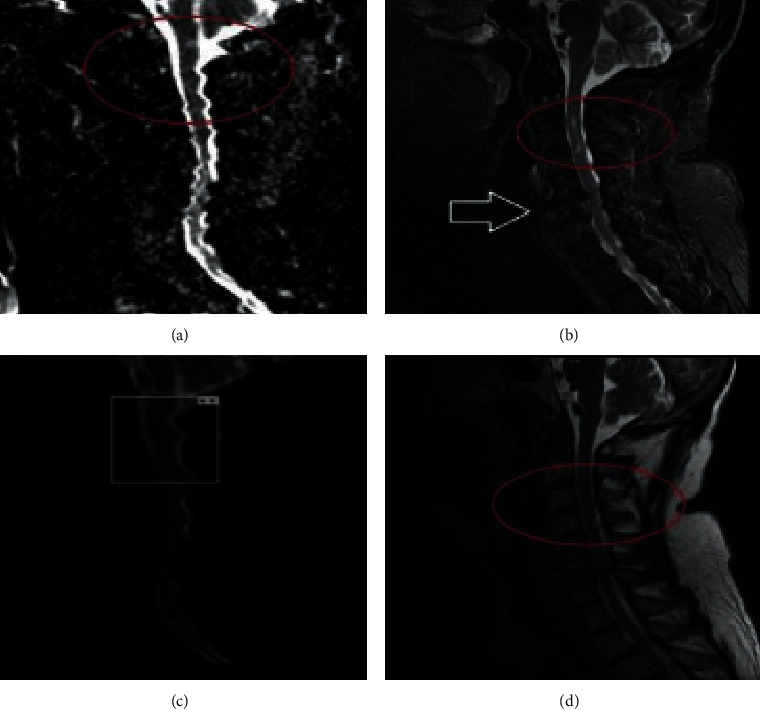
(a–d) MRI of the cervical spine with and without gadolinium demonstrated a restriction on diffusion-weighted imaging (DWI) (a) corresponding to the signal abnormality foci seen on short-TI inversion recovery (STIR) images (red circles in (b) and (d) and white marker image in (c)) and anterior compression from displaced cervical discs (white arrow in (b)).
